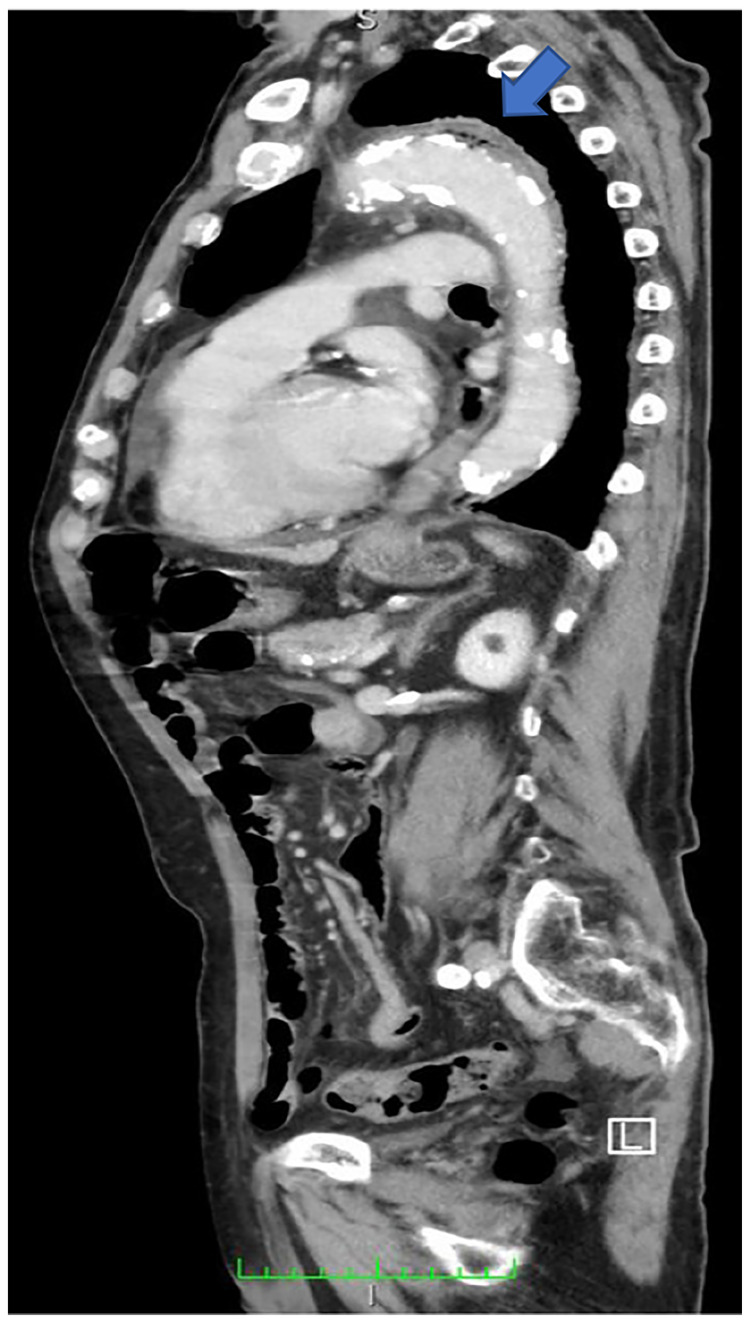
Chest computed tomography (sagittal view) showing emphysematous aortitis.
